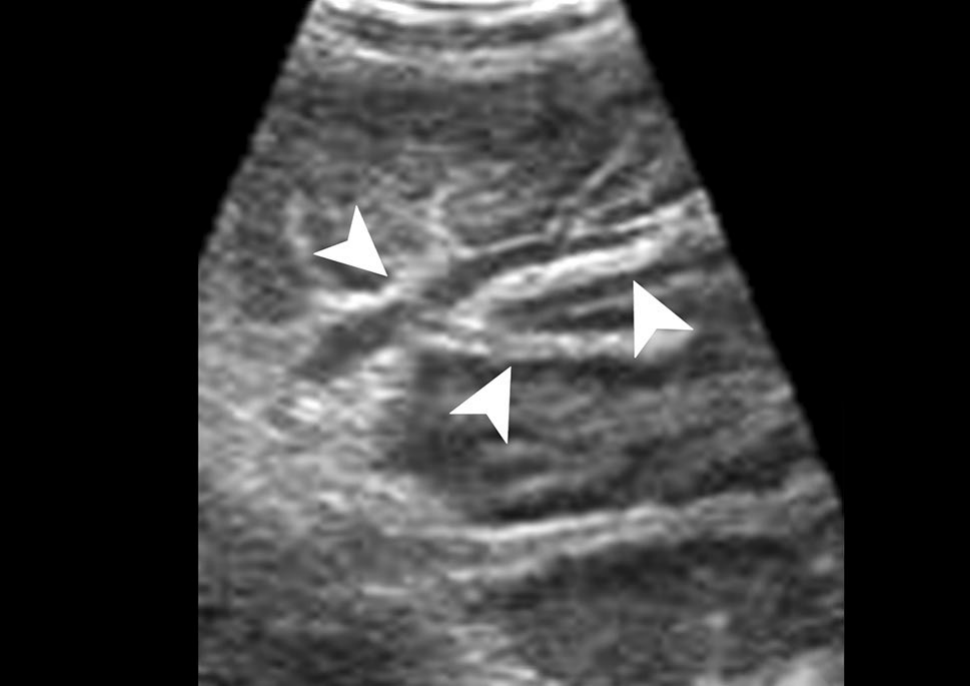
Hepatic schistosomiasis in a 20-year-old male patient with a history of gastrointestinal bleeding from 1 year ago. Ultrasound demonstrates a marked diffuse periportal thickening as a hyperechoic mantle encompassing the anechoic portal vein (white arrowheads) (Courtesy of Dr. Suzan Elhakiem, Ibn Sina Hospital, Khartoum, Sudan)
